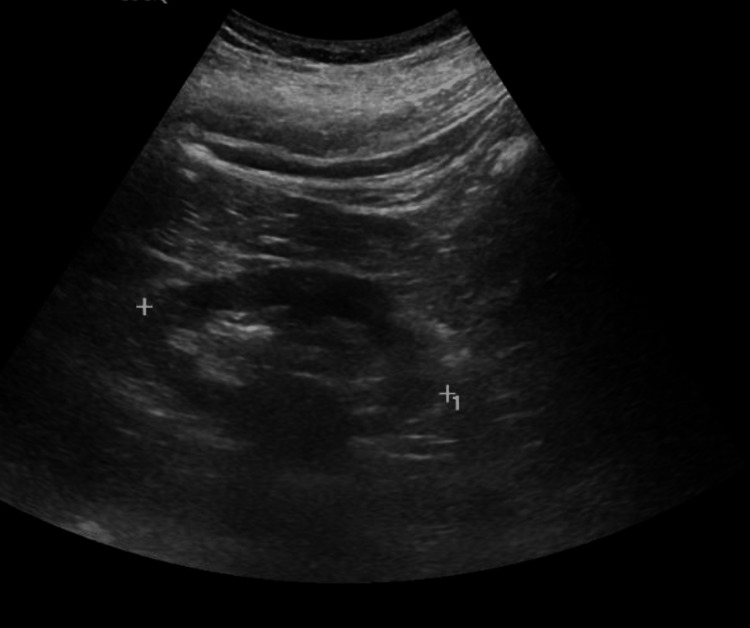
Ultrasound of the left kidney. Grayscale ultrasound image of the left kidney demonstrating grade II increased echogenicity.
